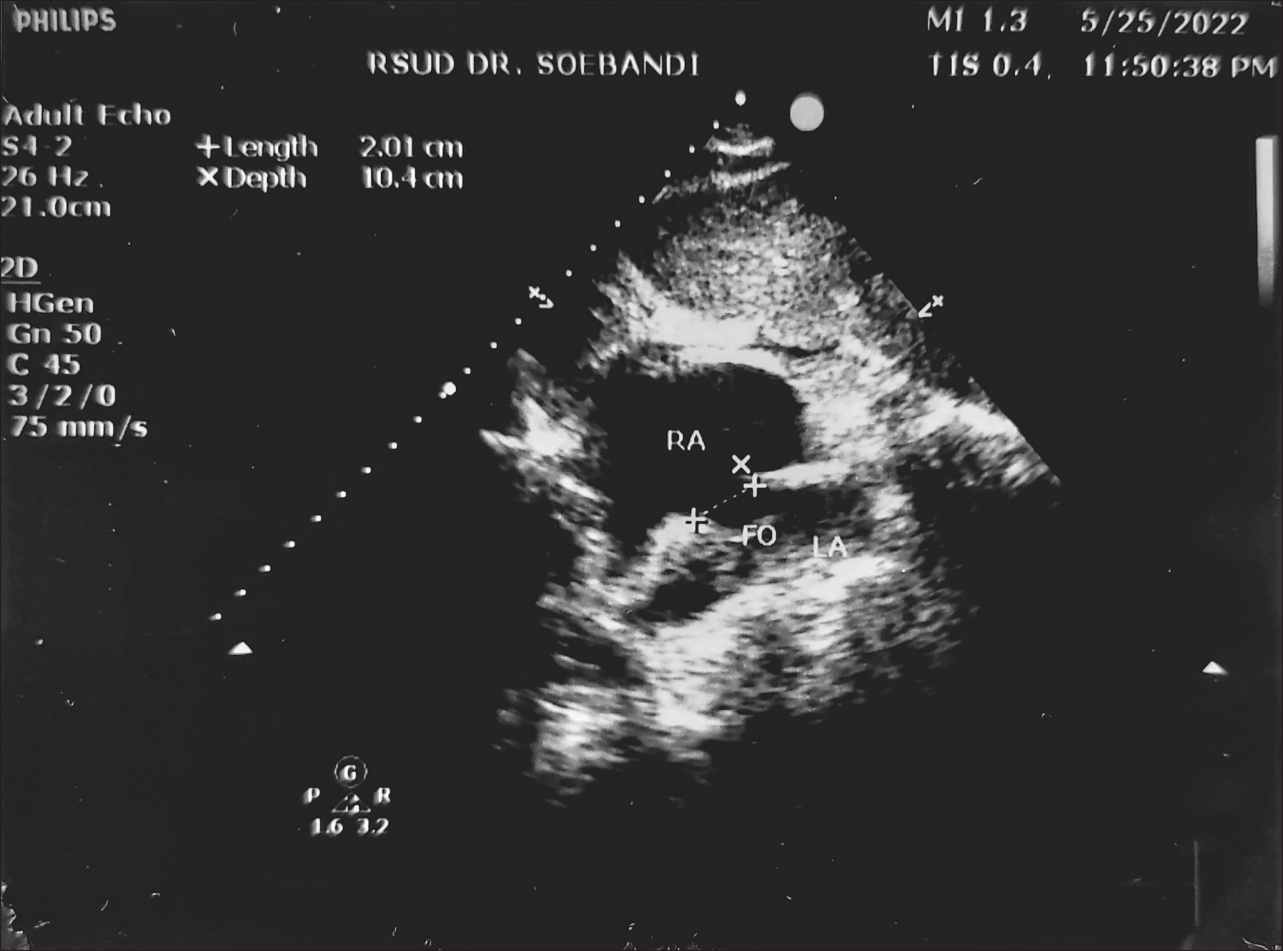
Emergency transthoracic echocardiogram. There was a 2-cm flap-like opening indicating patent foramen ovale.
